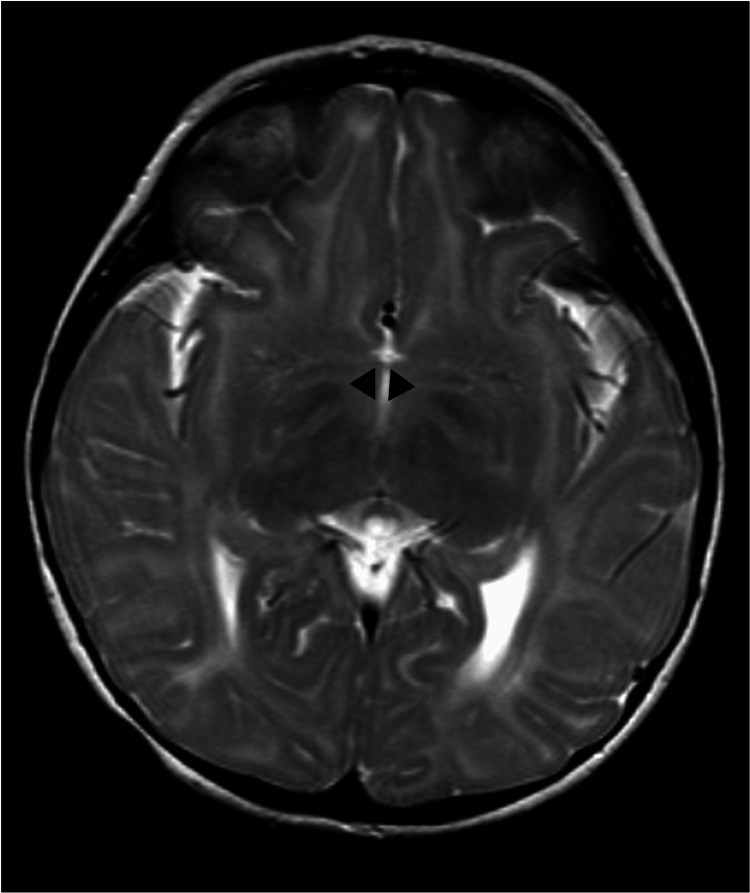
Axial T2 Weighted Imaging Axial T2-weighted image at the level of basal ganglia depicts subtle T2 hyperintense signal within the medial medullary laminae separating the medial and lateral segments of the globi pallidi (arrowheads).
